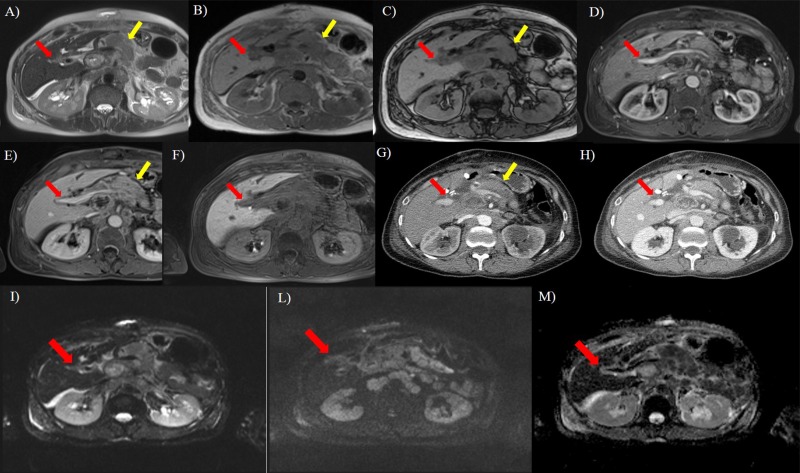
Seventy years old woman with pancreatic cancer (yellow arrow). In A (axial T2-W HASTE) the lesion shows (red arrow) hyperintense signal; in B (axial T1-W in phase) and C (axial T1-W out phase) it appears hypointense (red arrow). During contrast study (D, arterial phase, E, portal phase, F, hepatospecific phase) on MR, there is no evidence of the lesion so as on arterial (G) and portal (H) phase on CT study. DWI sequences: In I *b*50 s/mm^2^, in L *b*800 s/mm^2^, in M ADC map. The tumor (red arrow) shows restricted diffusion.
